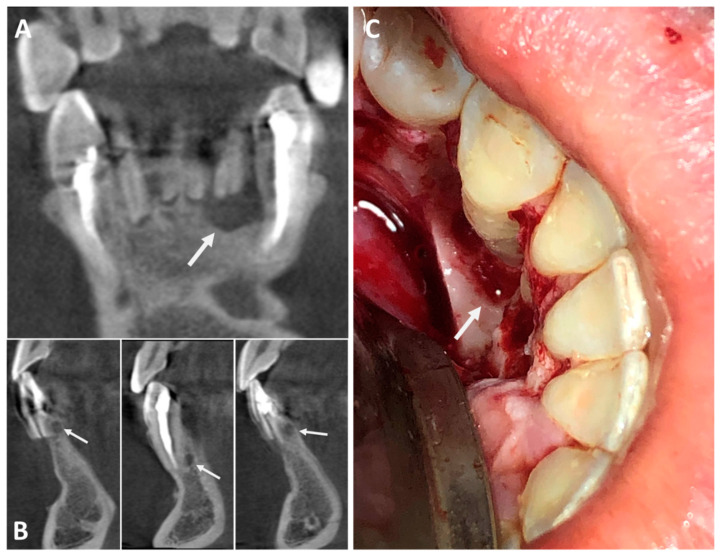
(A)—Representative coronal cut and (B)—sagittal cuts of the CT scan 8 months after the first surgery, revealing recurrent lesions in the apical region, lingually. (C)—Trans-operative image of the second surgical approach, aiming the removal of the recurrent lesion in the lingual portion of the cortex. The arrows point to the recurrent lesions.
